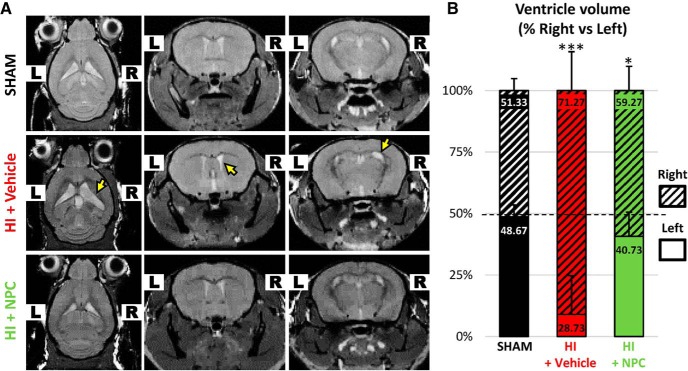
Transplanted NPCs help to recover the ventriculomegaly. *A*, MRI shows right unilateral ventriculomegaly after HI injury (arrow) on axial sections (left column), coronal sections at the anterior brain level (middle column), and coronal sections at the posterior brain level (right column). *B*, The percentage of right (dashed) versus left (plain) lateral ventricle showed a significant enlargement after HI (in red) compared to sham (in black). After NPC transplantation (in green) the brain structures, including the ventricle size, were partially restored (*A* and *B*). p* < 0.05, *p* < 0.01, p* < 0.001.
